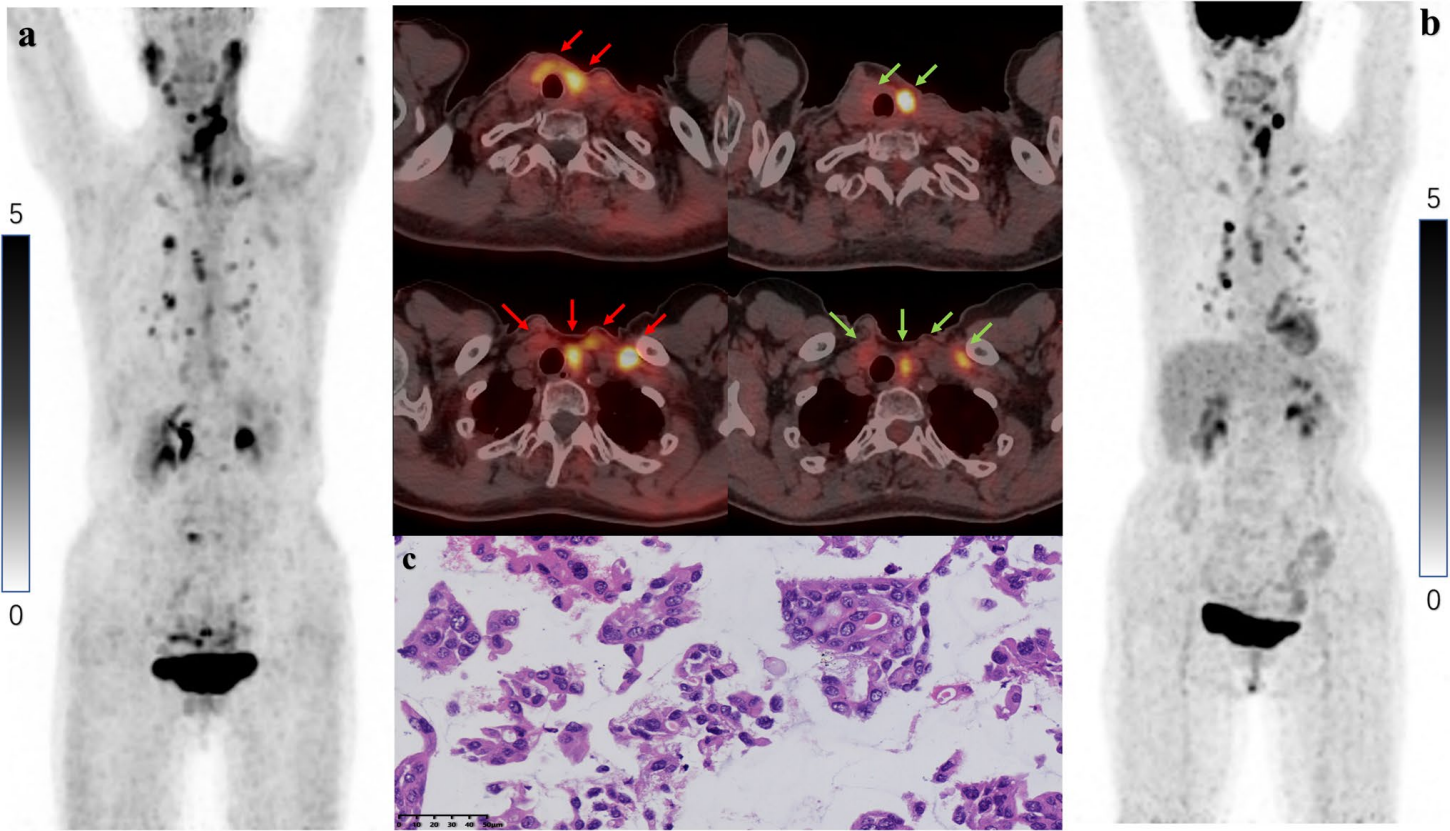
Local recurrences and metastatic lesions in a 66-year-old woman. a [^18^F]FAPI-42 PET/CT revealed local recurrence and metastatic lesions (in the lymph node and lung) with moderate-to-intense uptake (lesions are indicated by red arrows on axial fusion images). b 2-[^18^F]FDG PET/CT revealed fewer lesions and considerably lower radiotracer uptake than [^18^F]FAPI-42 PET/CT (lesions are indicated by green arrows on axial fusion images). c Pathologically confirmed diagnosis of PTC via US-FNA
